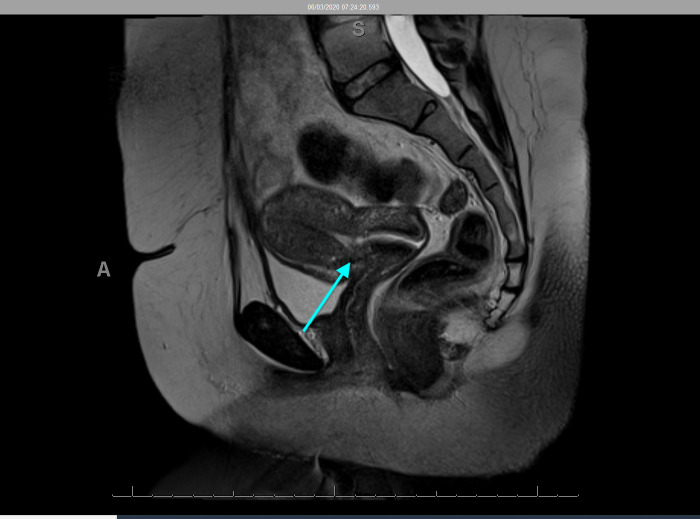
Case 1 Sagittal section of MRI demonstrating a possible niche and or fistula between the uterus and peritoneal cavity.
